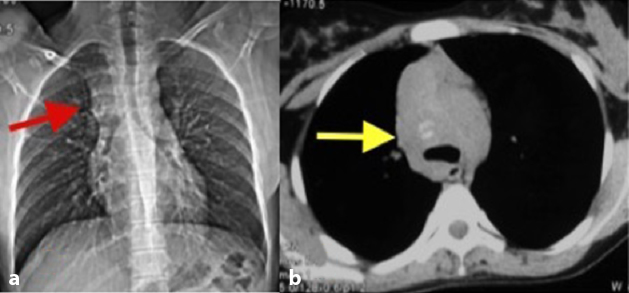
Chest radiograph (a) reveals widening of the superior mediastinum (arrow) and an axial non-contrast CT scan of the chest (b) in the mediastinal window shows an ill-defined mass with faint amorphous calcification (arrow).
